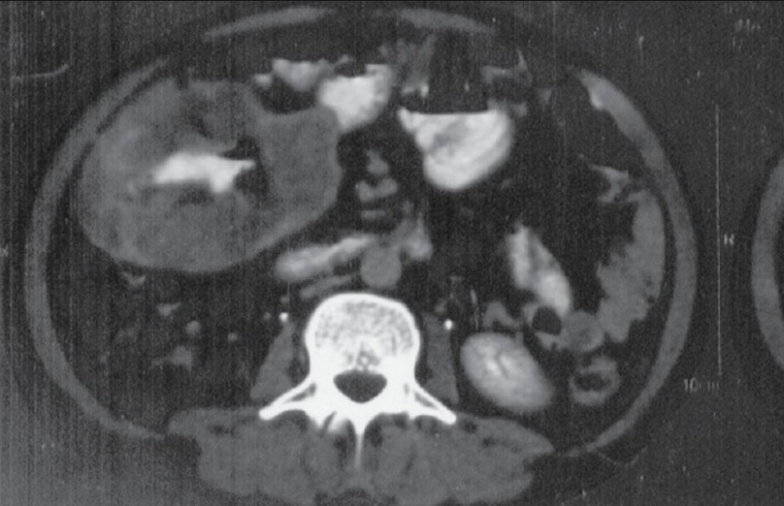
CT scan showing the lesion involving the small intestine
